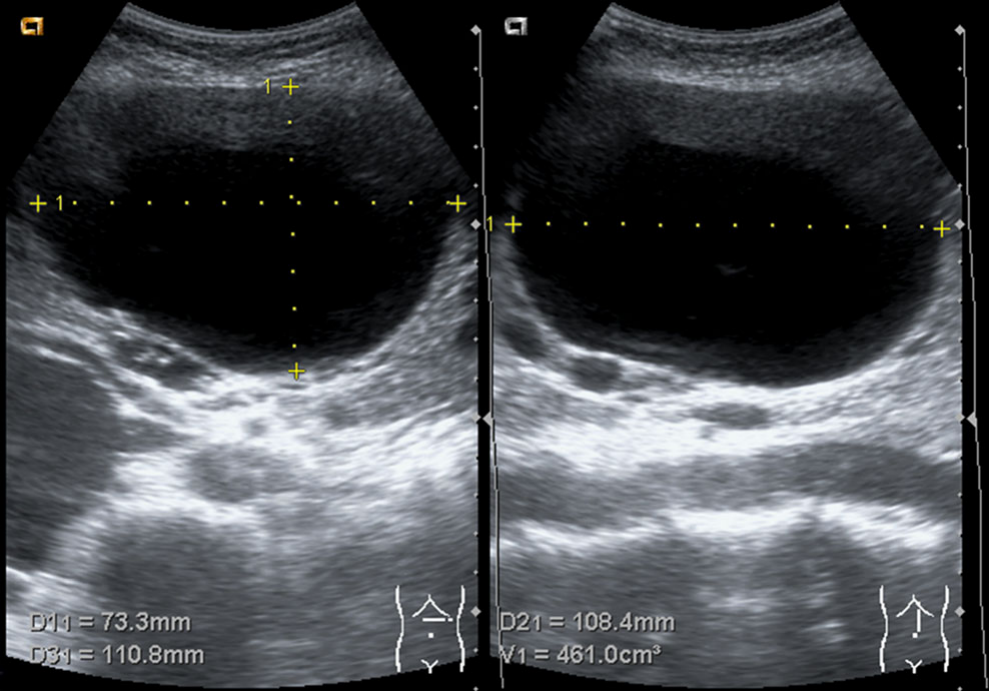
Hepatic cyst diameter measurement by ultrasonography; transversal (left) and coronal (right) plane to estimate hepatic cyst volume
